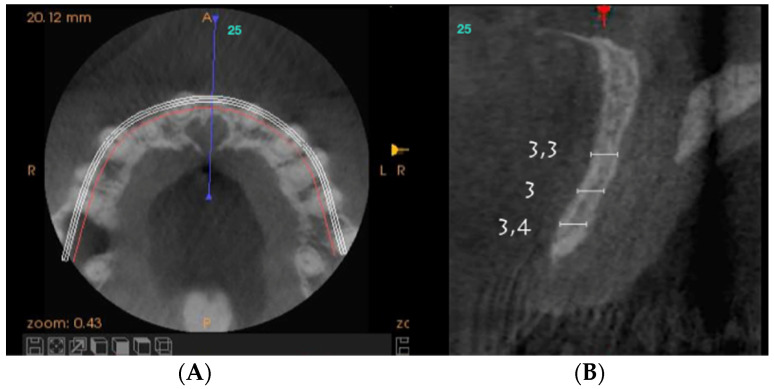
Representative sections of a Cone Beam Computed Tomography (CBCT) scan in which the bone thickness of the maxillofacial region and the widening of the incisive foramen are observed. (A) Occlusal view. (B) Sagittal view.
